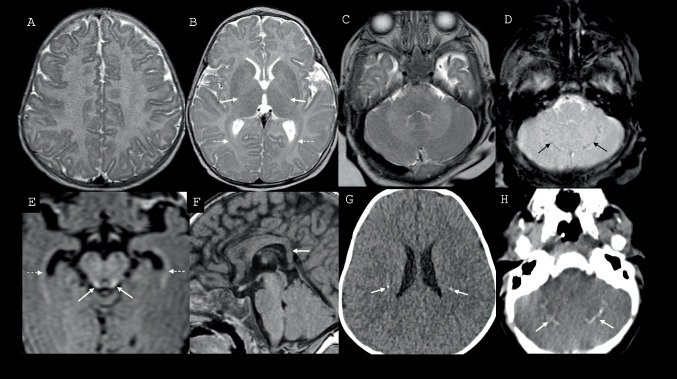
Brain MRI and CT findings at 4.5 months of life A-C: Axial T2-weighted images at the level of the centrum semiovale (A), deep gray nuclei (B) and posterior fossa (C) show diffuse abnormal hyperintensity consistent with deficient myelin deposition for age. Note relative sparing of the posterior limbs of the internal capsule (arrows, B), and optic radiations (dashed arrows, B) which demonstrate T2 hypointensity (also see E and F). D: Axial T2* weighted sequence shows symmetric curvilinear hypointensities involving the cerebellar white matter (arrows) consistent with calcifications (see G and H). E: Axial T1-weighted image shows myelination within the optic radiations (dashed arrows) and inferior colliculi (arrows). F: Sagittal T1-weighted sequence shows a thin posterior corpus callosum (arrow). G and H: Axial CT scans confirm punctate calcifications involving the posterior corona radiata (arrows, G), and curvilinear calcifications involving the cerebellar white matter (arrows, H)
